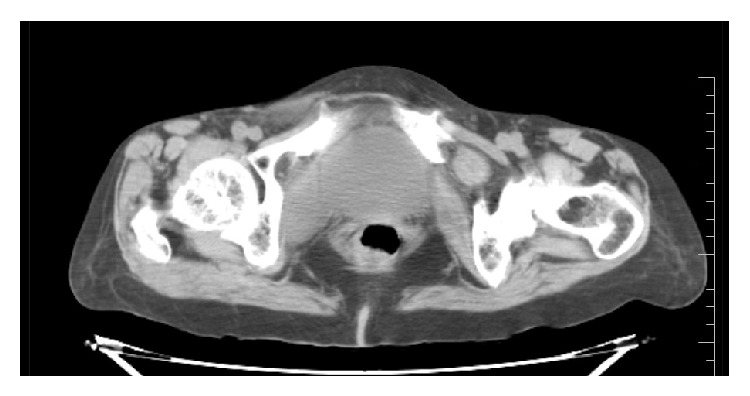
CT scan of lower pelvis and inguinal region showing herniation of small bowel loop through left obturator canal extending between pectineus and obturator internus muscles.
